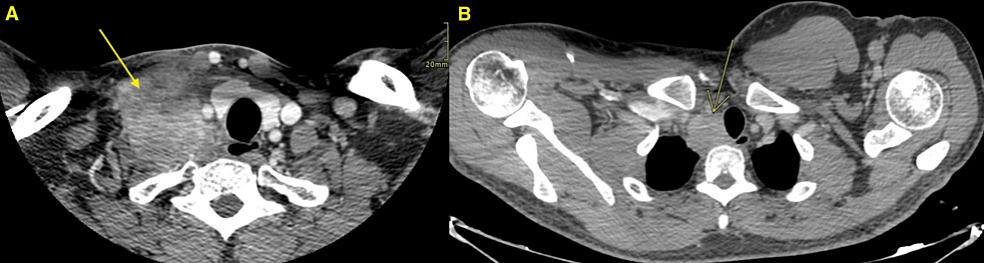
Chest CT The CT showed two right supraclavicular hypodense soft tissue masses; the larger one (A) measured about 5.4 x 5 x 5 cm at its anteroposterior (AP) x transverse (T) x craniocaudal (CC), respectively. The mass was seen abutting the thyroid gland and common carotid artery with the invasion of the proximal part of the internal jugular vein (IJV). The smaller mass (B) measured about 3.2 x 3.2 x 3.1 cm at its AP x T x CC, respectively. It encroached on the superior mediastinum pushing the trachea to the contralateral side and abutting the brachiocephalic artery CT: computed tomography
